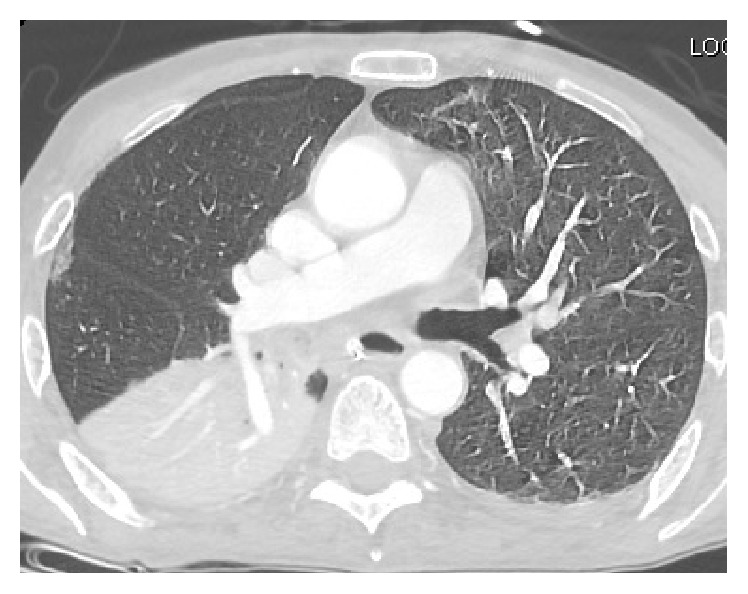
CT scan of the chest showing aspiration pneumonitis.
